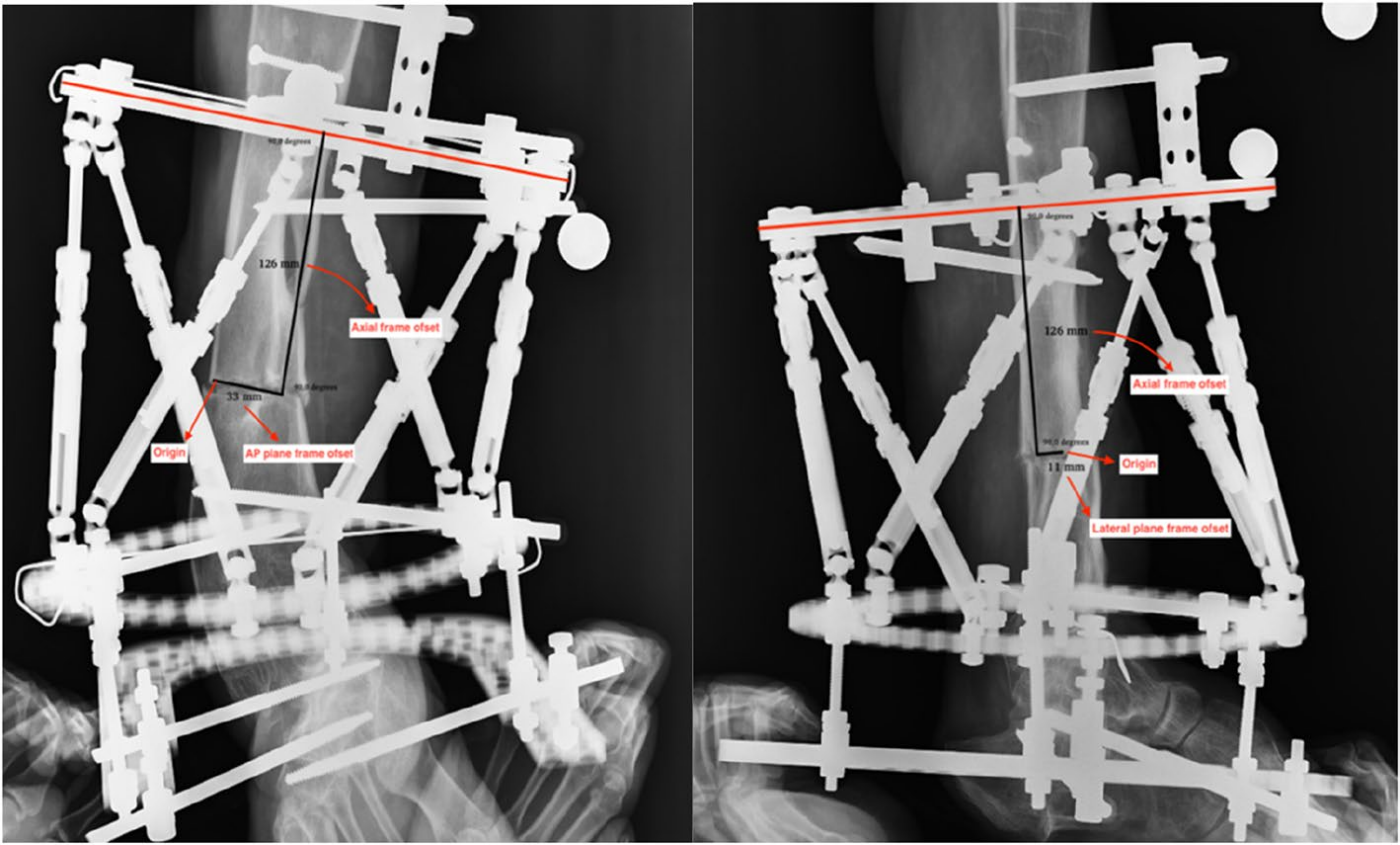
Mounting parameters shown on proximal reference radiograph of a tibial deformity
